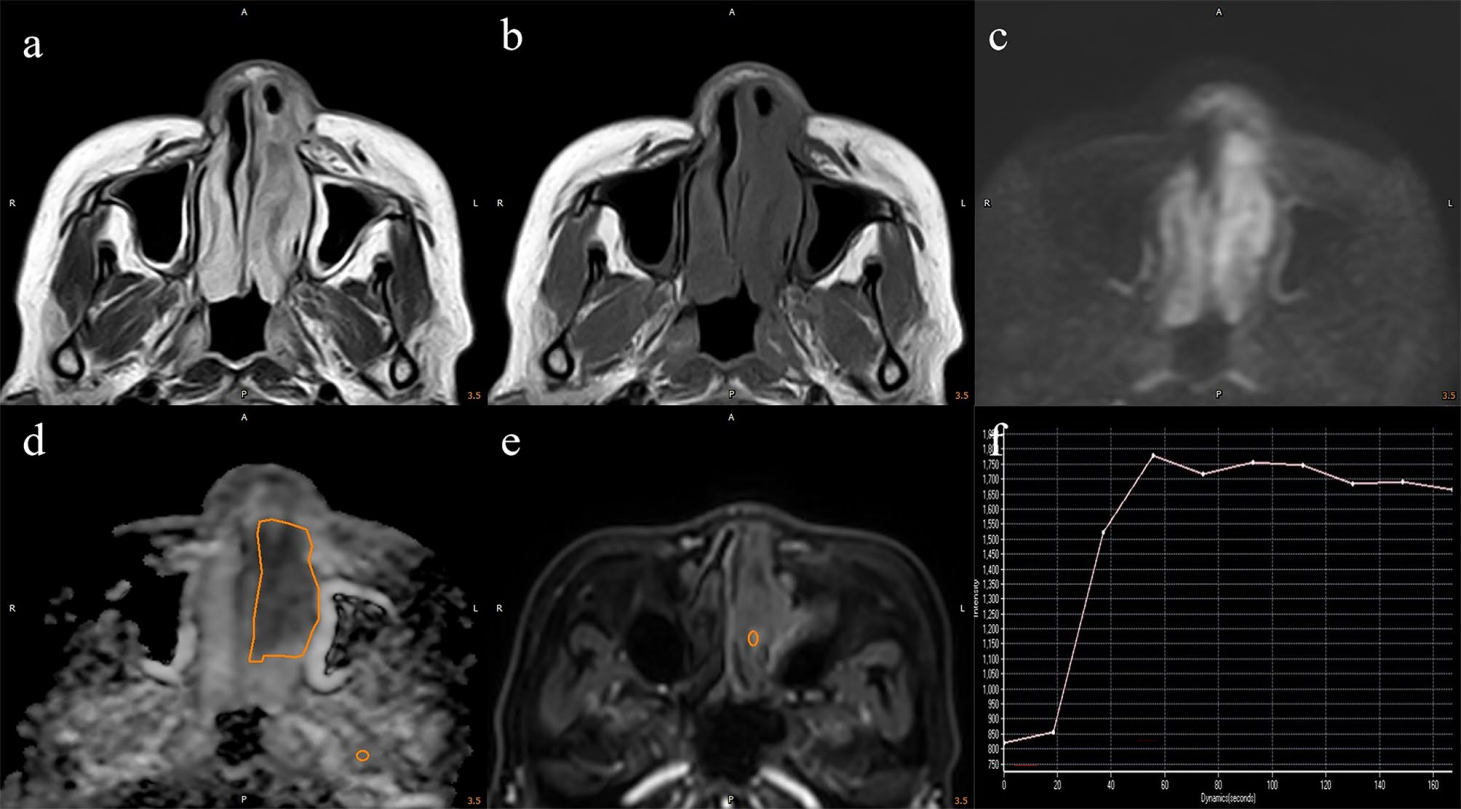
A 57-year-old female patient with sinonasal lymphoma, presenting in the left nasal cavity with relatively uniform signals of slightly high T2WI (a) and slightly low T1WI (b). The DWI (c) demonstrates high signal intensity, while the ADC map (d) shows decreased signal. The yellow lines in images d and e represent the region of interest (ROI) measurement method. Image f displays a type II time-intensity curve (TIC)
